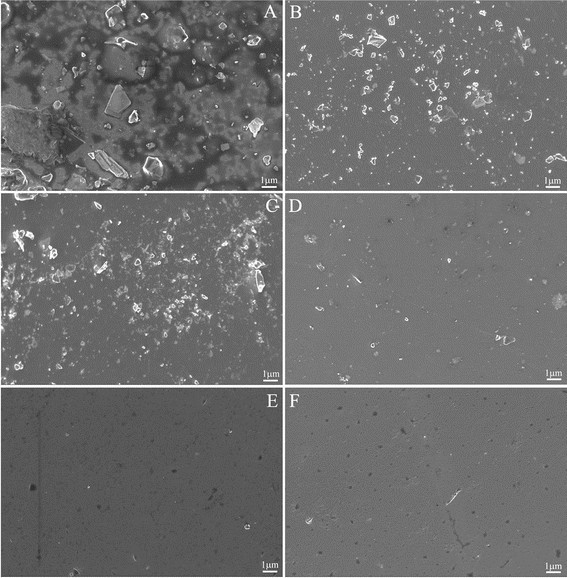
Surface properties observed by SEM. Surface of untreated pyrite (A), pyrite after 15 minutes in boiling HCl (B), pyrite after pretreatment by Method 1 (C), and pyrite after the 3^rd^(D), 6^th^(E) and 12^th^(F) ultrasonication cycle in Method 2 (see text for details).
